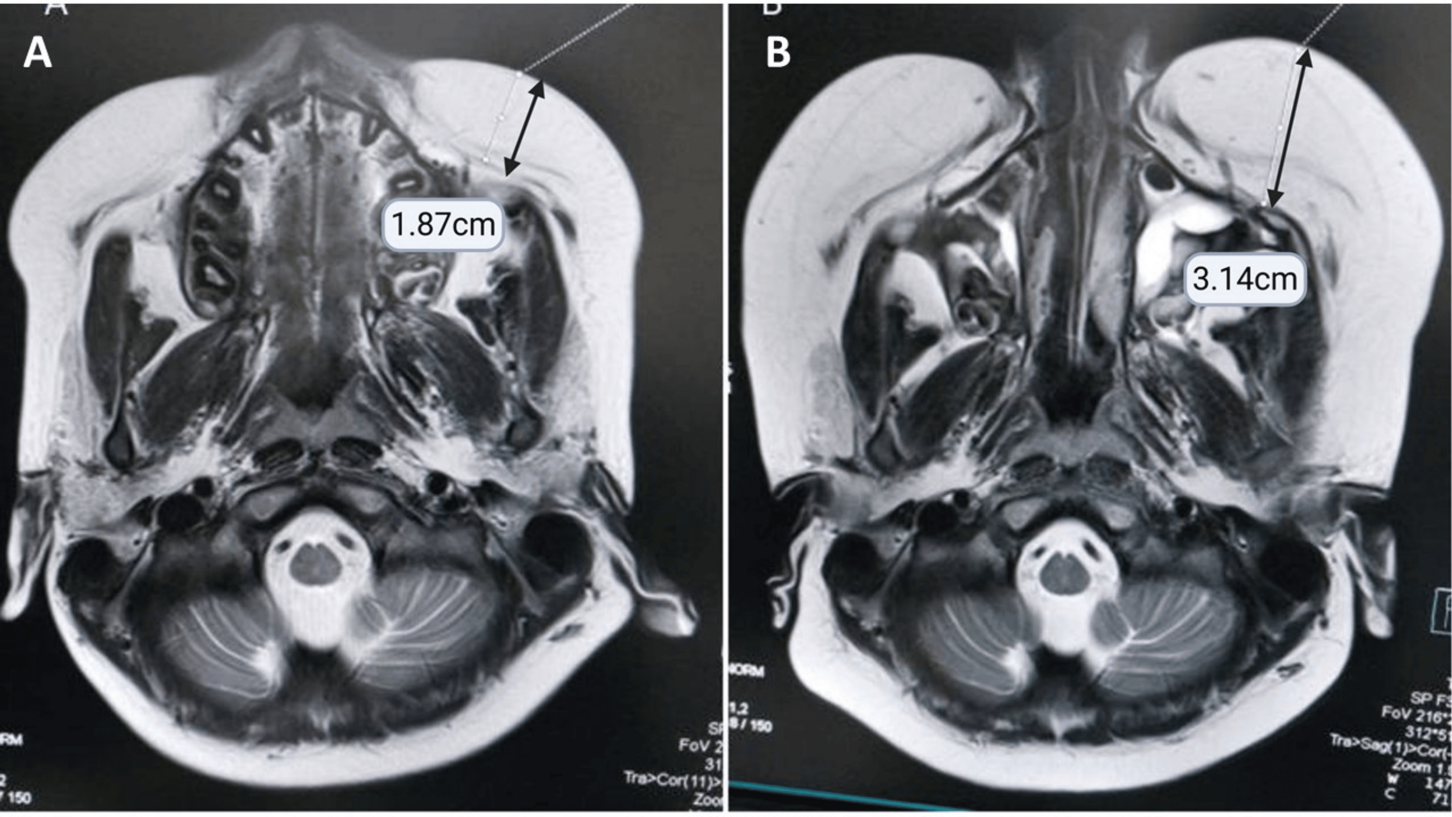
MRI brain showing premaxillary fat hypertrophy (A) T2W axial image of the face at the time of clinical presentation shows premaxillary fat thickness of 18 mm in the left maxillary region. (B) T2W axial image of the face at follow-up after 6 weeks of steroid therapy, at the same level corresponding to C, shows significant hypertrophy of the premaxillary fat, which measures at 32 mm. T2W: T2-weighted; MRI: magnetic resonance imaging
